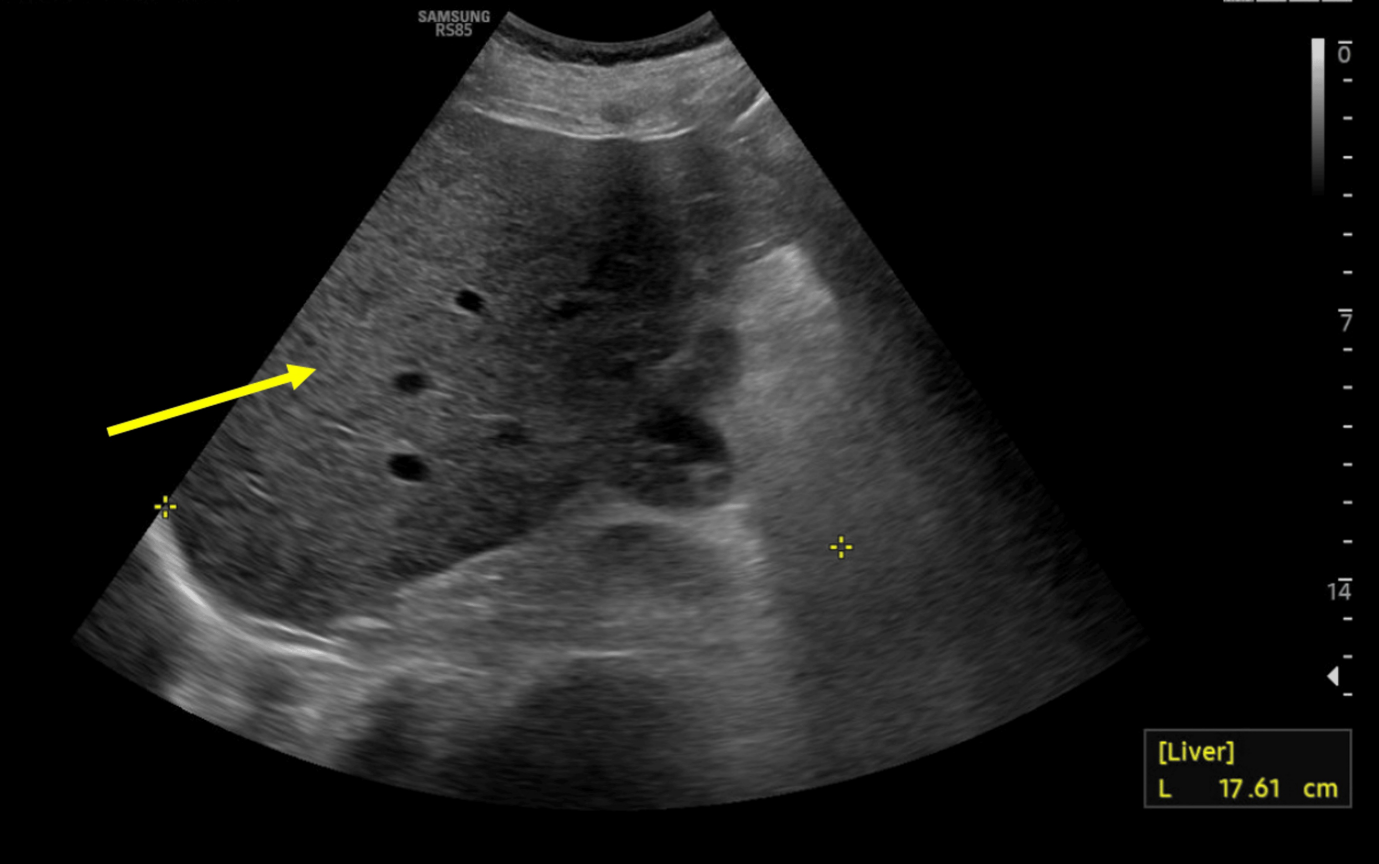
Liver ultrasound of the patient showing hepatomegaly with no biliary dilation Liver ultrasound shows hepatomegaly (right lobe 17.6 cm, see yellow arrow). The main portal vein has normal hepatopetal flow, ruling out portal vein thrombosis. No intrahepatic biliary dilation or ascites is present, supporting sinusoidal obstruction syndrome (SOS) as the cause of liver dysfunction.
